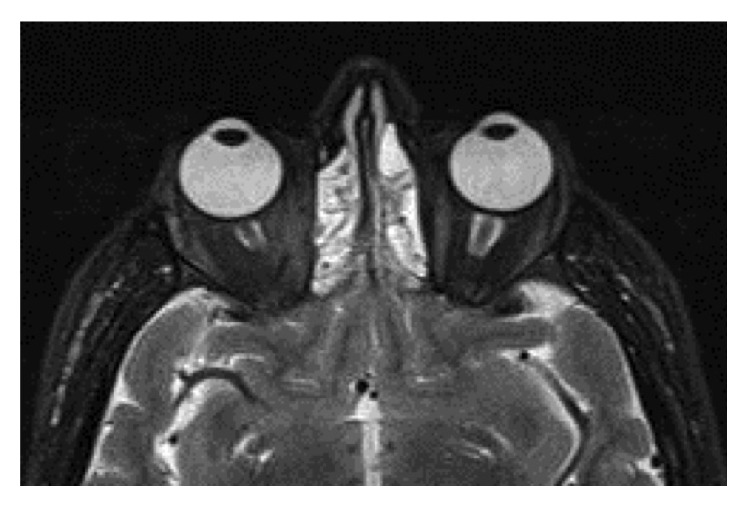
Orbital magnetic resonance imaging showed exophthalmos with enlargement of both eye muscles specially on the right.
